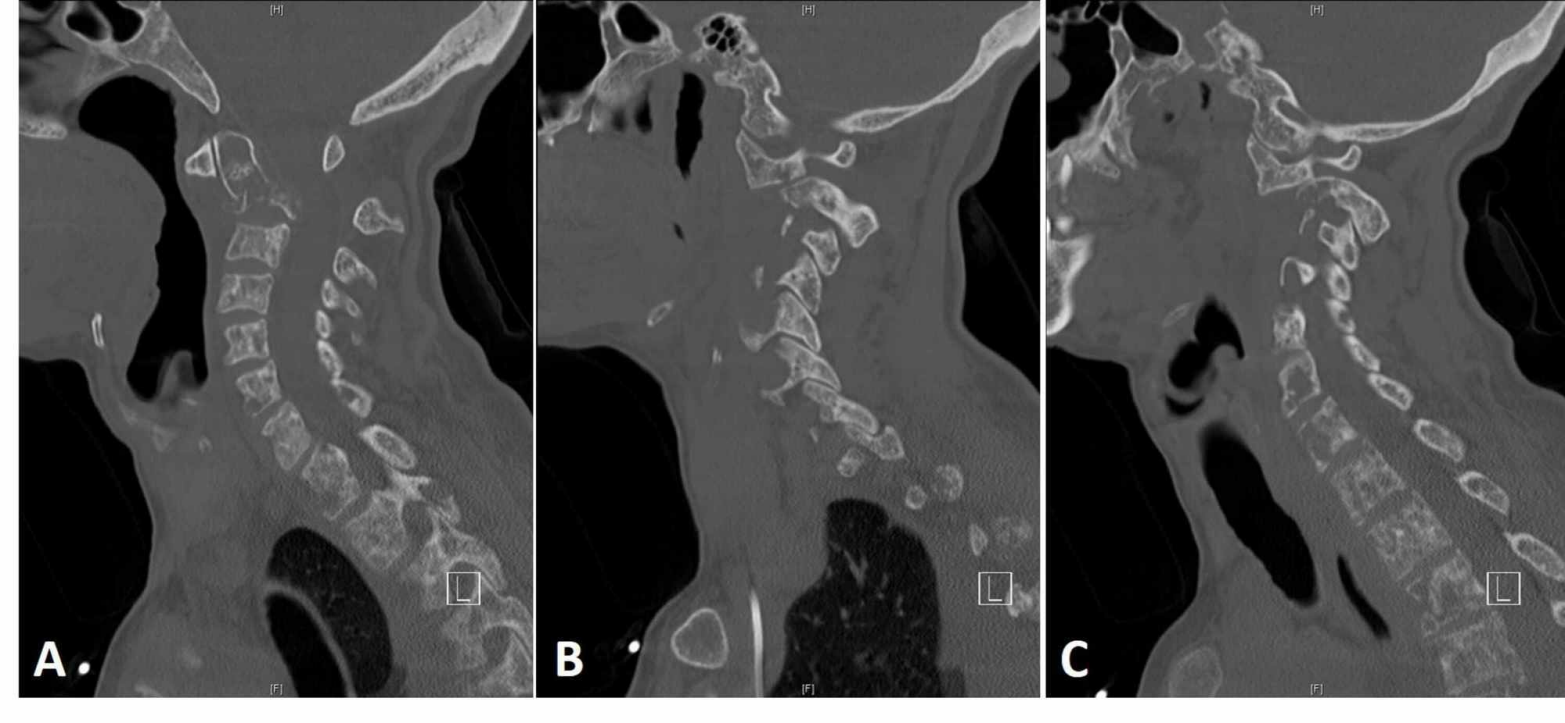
Preoperative CT of the cervical spine without contrast CT of the cervical spine without contrast showing acute, displaced, and impacted pathologic fracture of the base of the odontoid process extending into the C2 body (A mid-sagittal), anterior subluxation of the lateral mass of C1 on C2, and abnormal widening of the interval between the posterior arch of C1 and C2 spinous process (B left and C right parasagittal).
